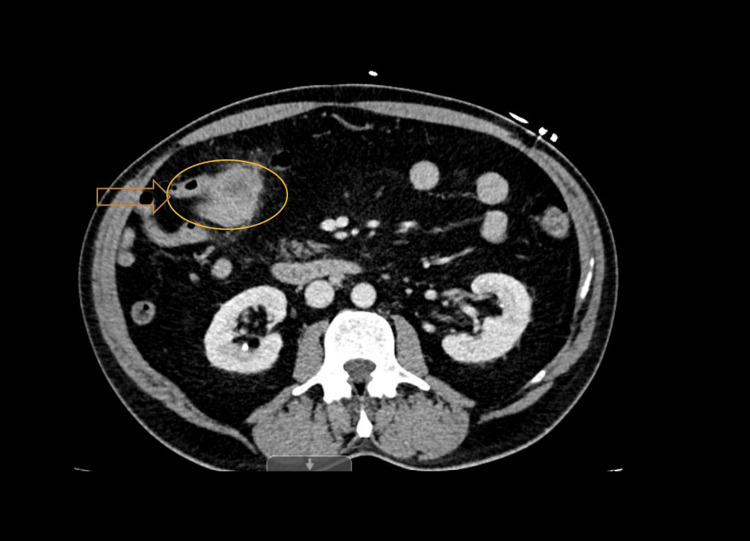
Abdominal CT scan post-contrast showing a 4.9 cm apple-core-like mass at the right-sided ileocolic anastomotic area concerning for primary or recurrent colon cancer
